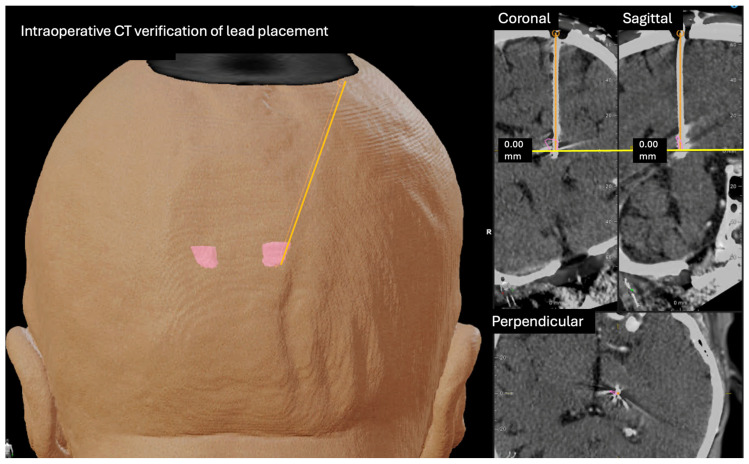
Postoperative verification of DBS lead placement. Fusion of preoperative magnetic resonance imaging with intraoperative computed tomography acquired using the Loop-X system demonstrates accurate electrode positioning along the planned trajectory and concordance with the intended VIM target. Axial, coronal, and sagittal views are shown.
